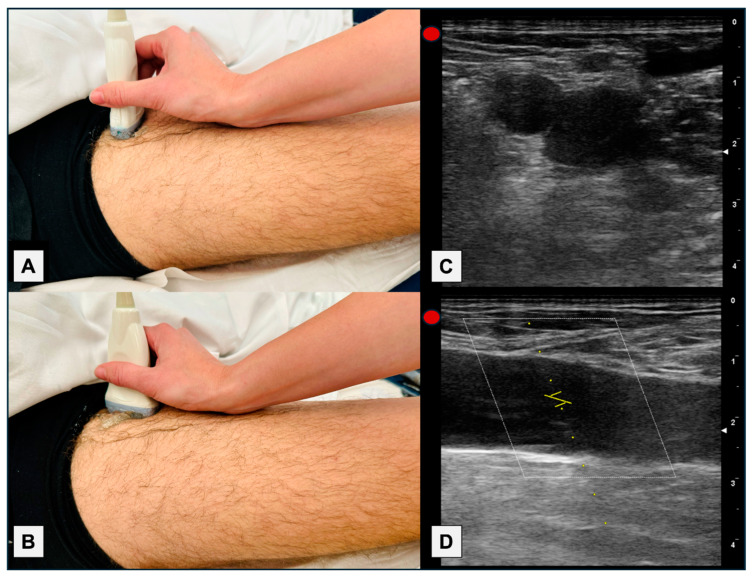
(A) Probe’s position on the patient for a transverse section. (B) Probe’s position on the patient for a longitudinal section. (C) Ultrasound image of the transverse section at the level of the CFV. (D) Ultrasound image of the longitudinal section of the right CFV. In this case, the cranial region is on the left (transducer mark) and the caudal region is on the right of the image.
